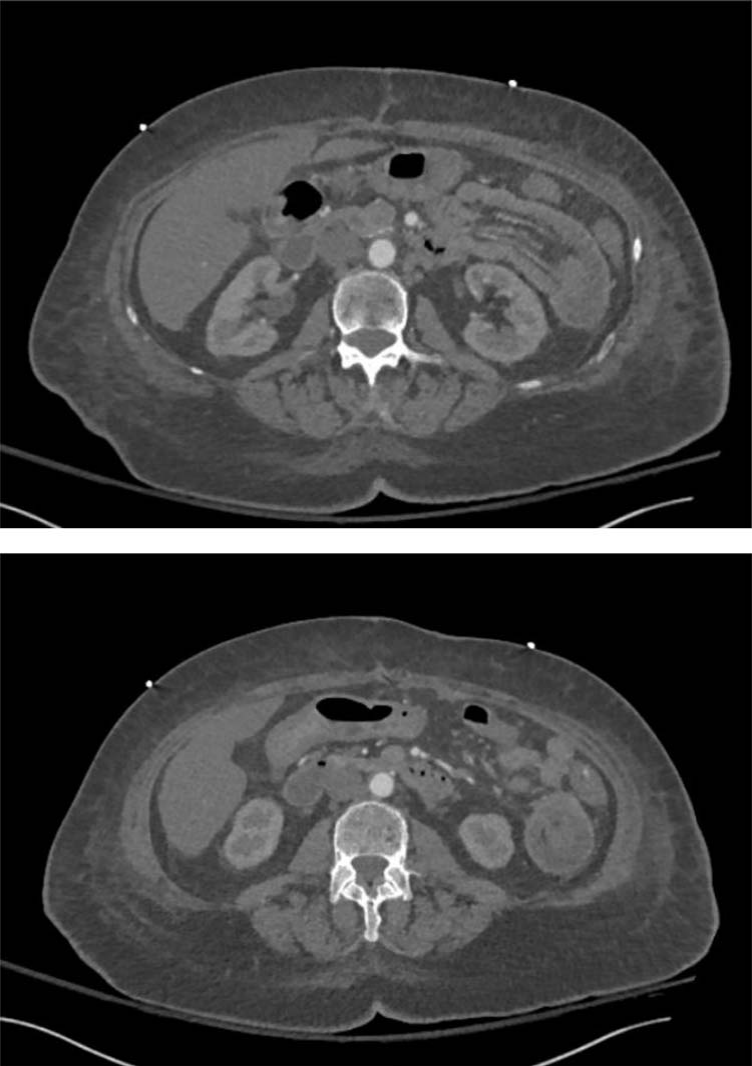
Intussusception of the proximal small bowel on CT abdomen without contrast.
